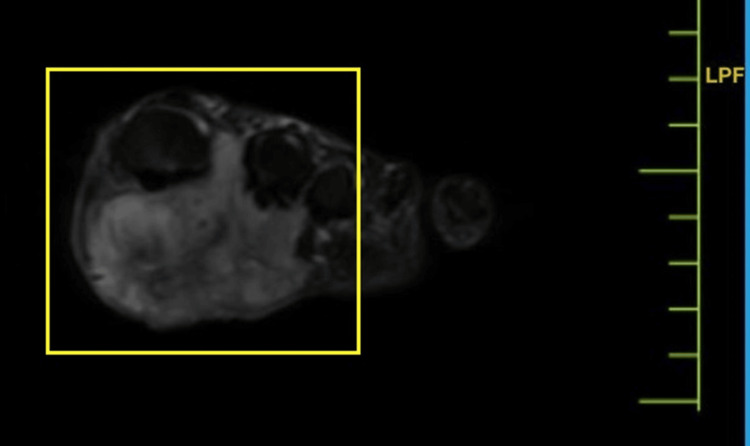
MRI of the foot (axial plane) MRI: magnetic resonance imaging
